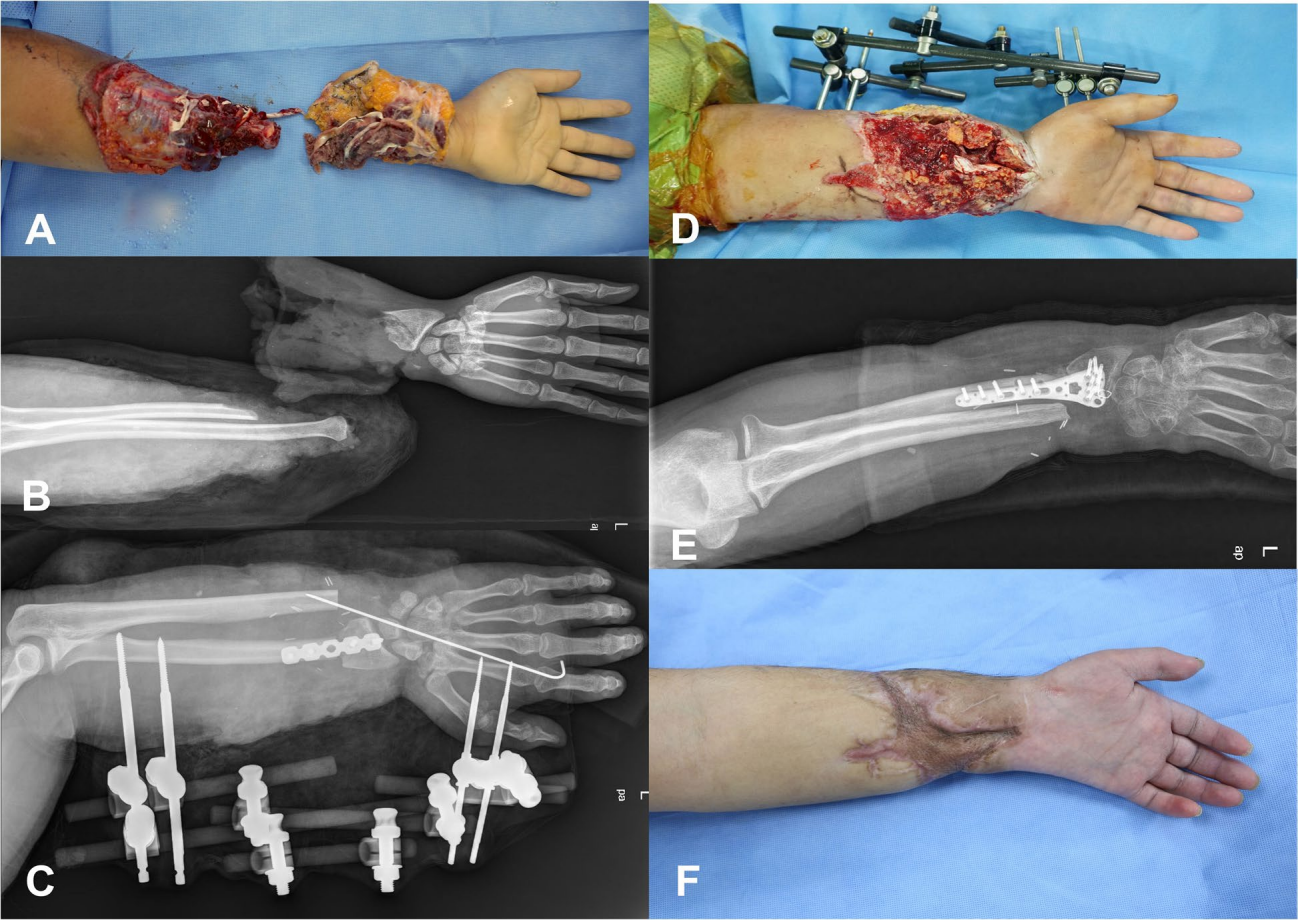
A A 23-year-old male patient experienced traumatic amputation of the middle of the forearm of the left arm due to a traffic accident. B The X-ray shows that the injury occurred at the distal radius and the ulnar bone is disarticulated. C We performed bone fixation to the distal radius with a 5-hole plate and performed resection of the distal ulna, followed by an external fixator. D Due to severe skin loss at the amputation site, a skin graft is planned for the subsequent surgery. E During the second surgery, we replaced the plate with a volar locking plate at the distal radius. F This photo was taken 3 months after the initial trauma, and subsequent rehabilitation therapy was performed
